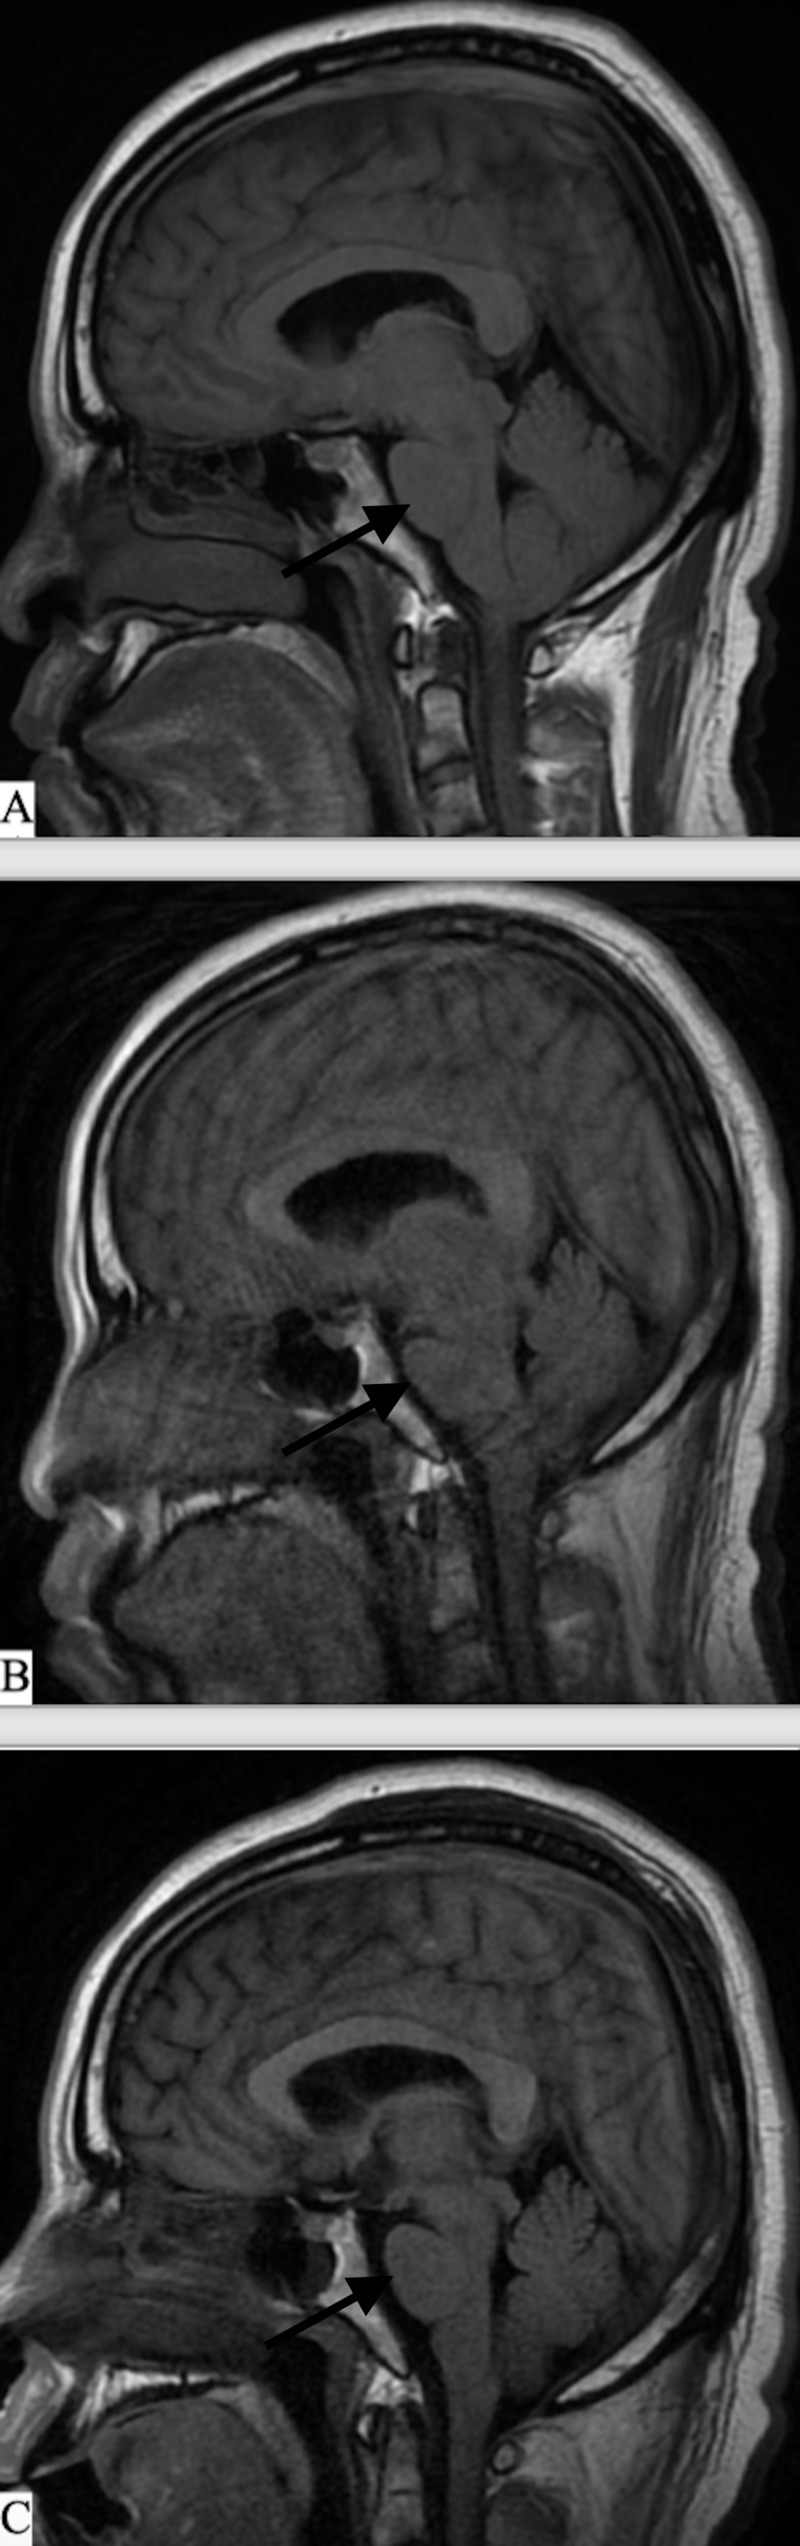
Pontomedullary junction on sequential MRI brain A) MRI brain with gadolinium (GAD), sagittal. MRI imaging during the initial workup. Re-demonstrated for comparison purposes. B) MRI brain without GAD, sagittal view. On admission with the start of altered mentation. Evidence of shortened pontomedullary distance. Prior to subdural drain placement. C) MRI brain without GAD, sagittal view. After the removal of subdural drains. Persistent bed rest and hydration. Improvement in the pontomedullary distance.
